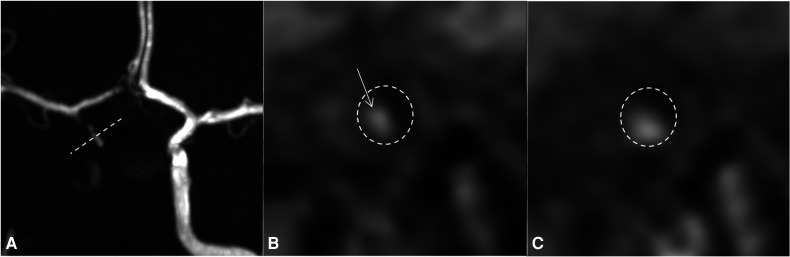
Day 4 MRI with TOF sequence (A) and 3D SPACE T1 imaging of the vessel wall before (B) and after gadolinium injection (C). The reconstruction of (B) and (C) images follows the dotted line on the TOF sequence (A: dotted line). For easy identification, the internal carotid artery is surrounded by a dotted circle on (B) and (C). On the TOF sequence, the right internal carotid artery is occluded and the right anterior circulation is supplied downstream by the right PComm and AComm. On vessel wall imaging, there is a spontaneous marginalized and asymmetric hyperintense signal (B, arrow) that enhances after injection of gadolinium, without enhancement of the vessel wall itself (C), highly indicative of a wall hematoma due to focal intradural dissection of the right internal carotid artery. MRI, magnetic resonance imaging; PComm, posterior communicating artery; AComm, anterior communicating artery.
